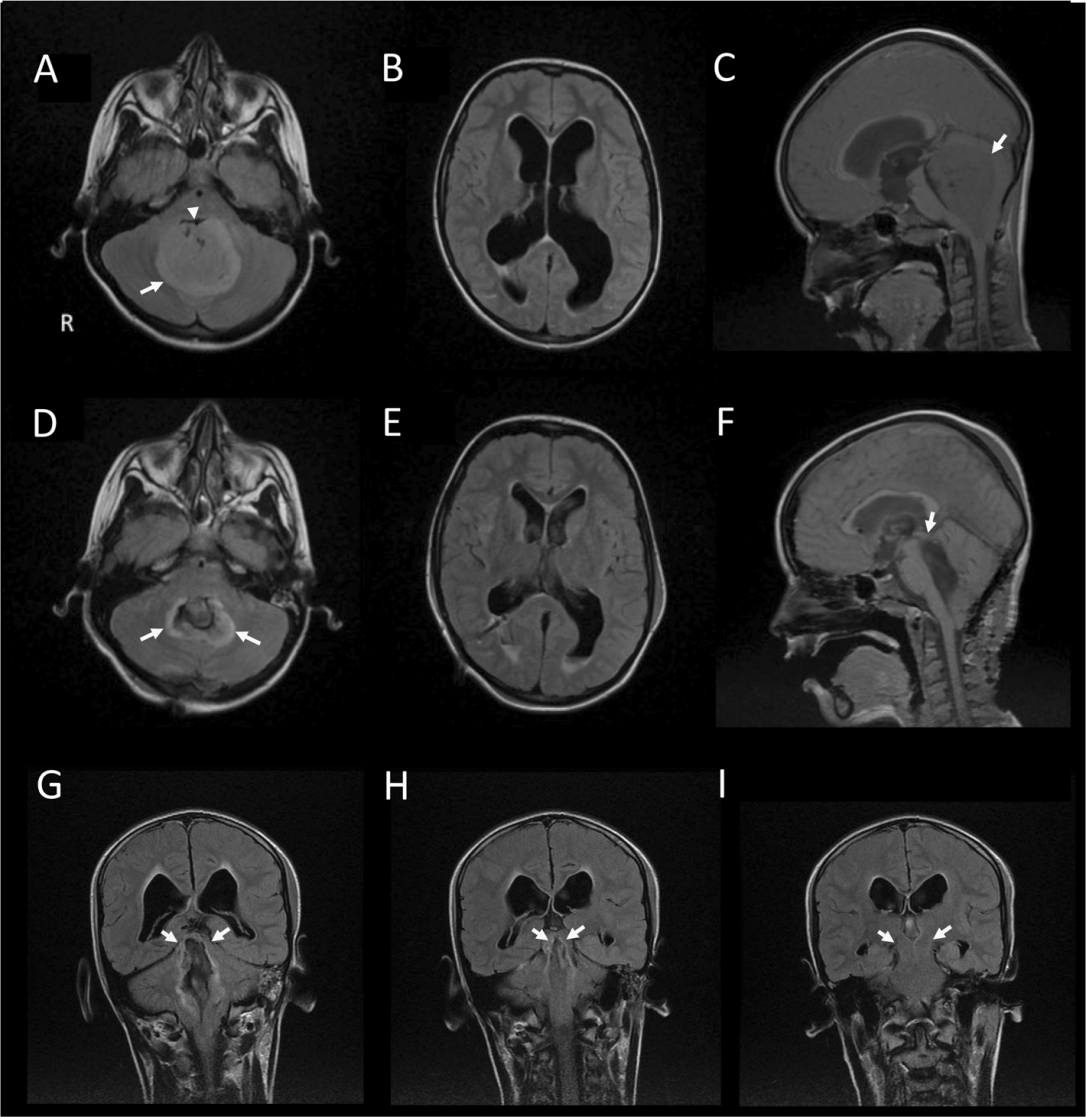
(a-i) Preoperative brain MRI (a-c). The *white arrow* (a; axial Flair sequence) points to the tumor invading the cerebellar parenchyma bilaterally. The lesion appears slightly hyperintense. The 4th ventricle is invaded (*white arrowhead*), causing a hydrocephalus (dilatation of the lateral ventricles in b). The tumor expands clearly in the vermis as shown on a sagittal image (c). Early postoperative axial FLAIR slice shows postoperative sequelae at the level of dentate nuclei bilaterally (*white arrows* in d). The hydrocephalus is resolved (e). The 4th ventricle is moderately dilated, including in the rostral direction. The superior medullary velum is visible (*white arrow* in f). The superior cerebellar peduncles are involved (*white arrows* in g), with a slight extension towards the corpora quadrigemina at the level of inferior colliculi (*white arrows* in h). Crus cerebri are spared bilaterally (*white arrows* in i). R: right side
